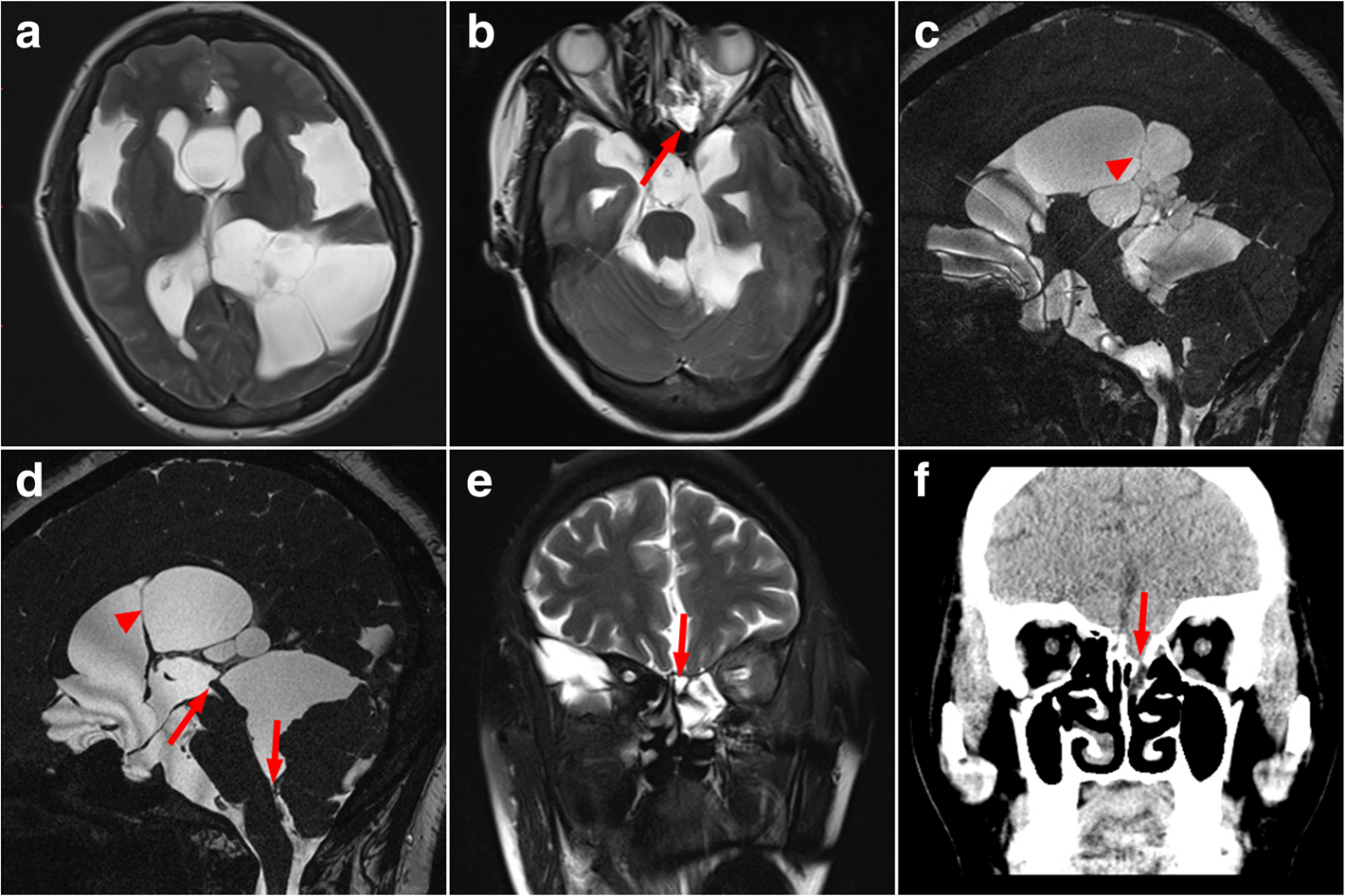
Preoperative MR image. a and b The T2-weighted axial image: multiple arachnoid cysts. c and d Preoperative Cine phase-contrast MR image: Arrow directs that the aqueduct of sylvius and the exit of fourth ventricle are blocked. e The T2-weighted coronal MR image: Arrow shows that the left anterior cranial fossa is likely communicated with the left ethmoid sinus. f A coronal high-resolution bone window noncontrast CT image: Arrow indicates that left ethmoid is discontinuous
